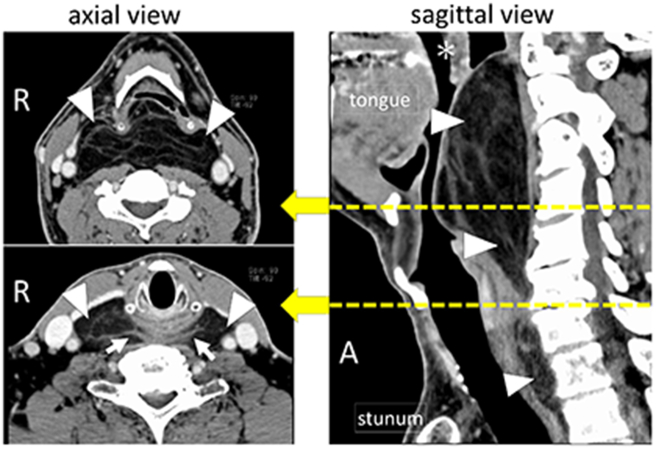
Computed tomography scanning. Axial view: The tumor in the retropharyngeal space (arrowhead). The septum-like structures were prominent behind larynx (arrow). Asterisks(*):uvula. Sagittal view: The tumor of retropharyngeal space was extended from soft plate to mediastinum (arrowhead).
